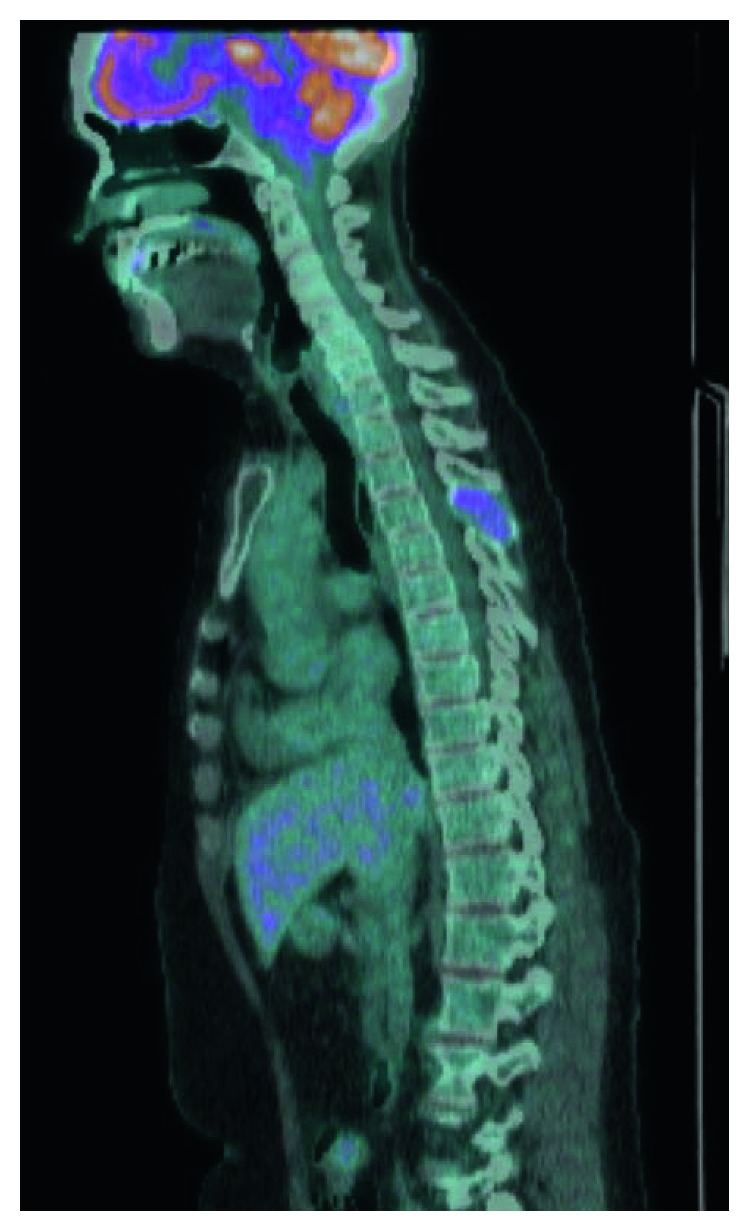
Positron emission tomography (PET). PET scan displayed a fluorodeoxyglucose (FDG)-avid lytic lesion in the left skull base where the previously noted mass on MRI was seen, as well as FDG uptake within the previously noted T4 lesion, seen here as the purple coloration within the T4 vertebral body.
